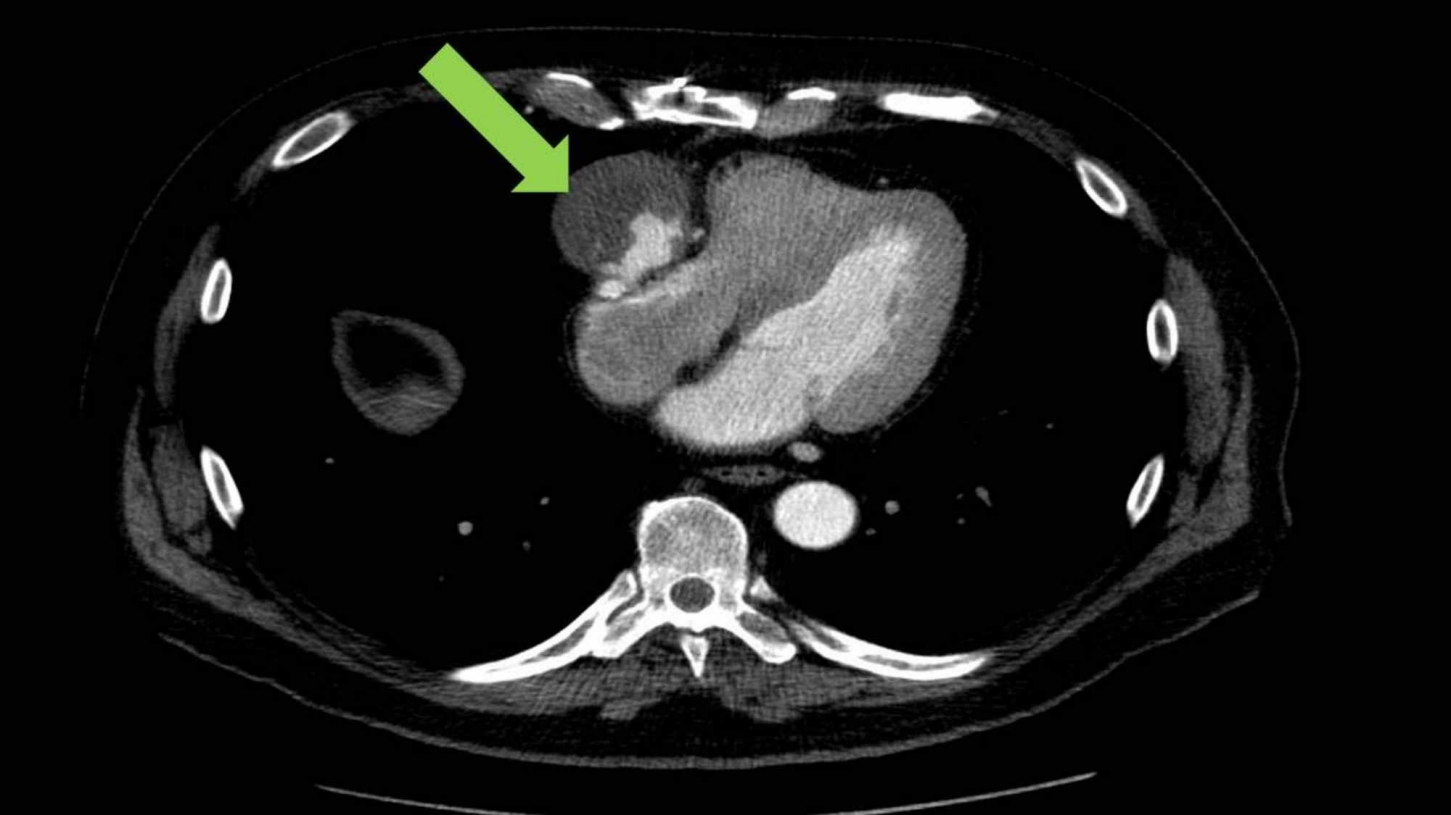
CT angiogram at diagnosis Green arrow indicates a pseudoaneurysm
